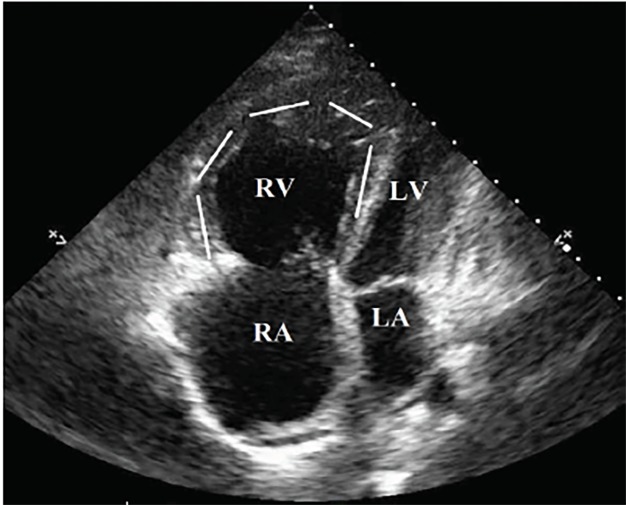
Transthoracic echocardiogram apical view demonstrating severe right ventricular (RV, walls outlined) and right atrial (RA) dilation consistent with right heart strain. *LV,* left ventricle; *LA*, left atrium.
